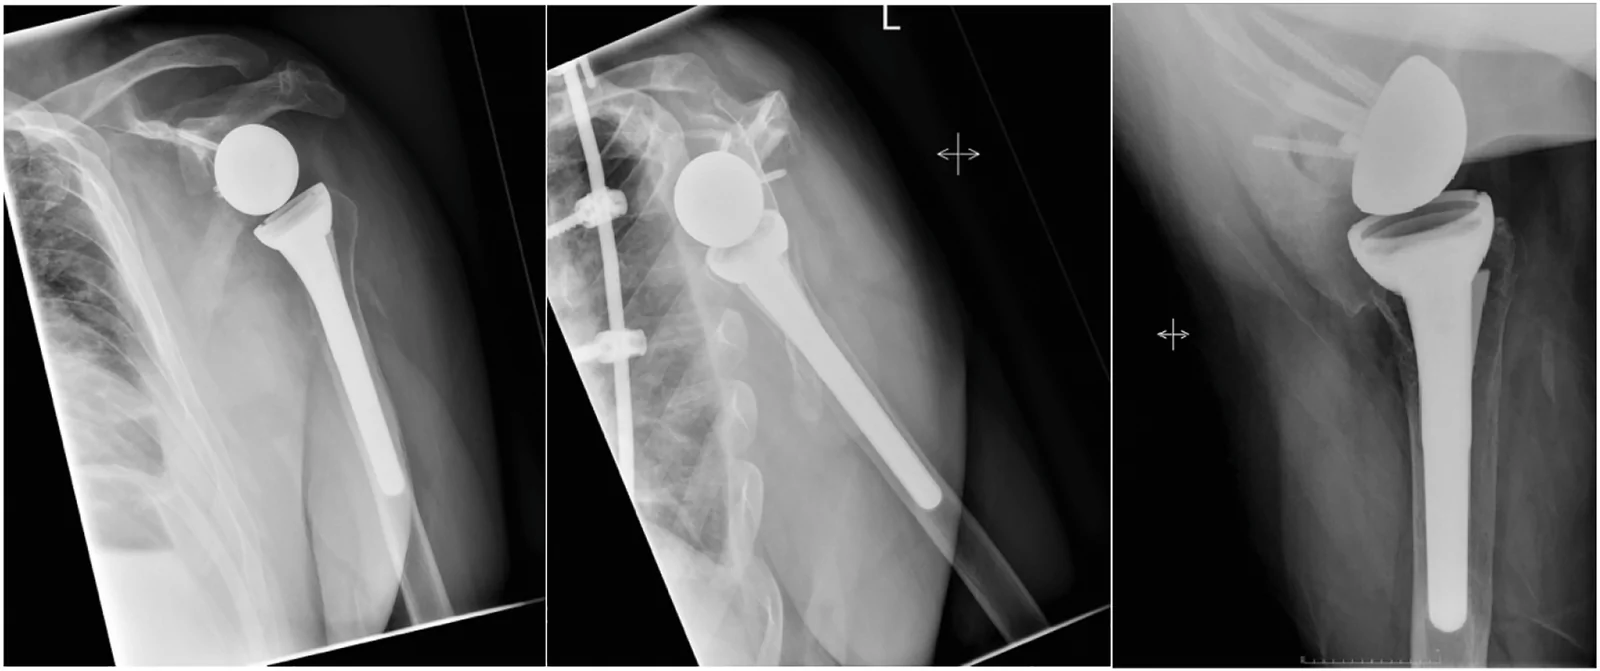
Case 2 post revision x-rays.
